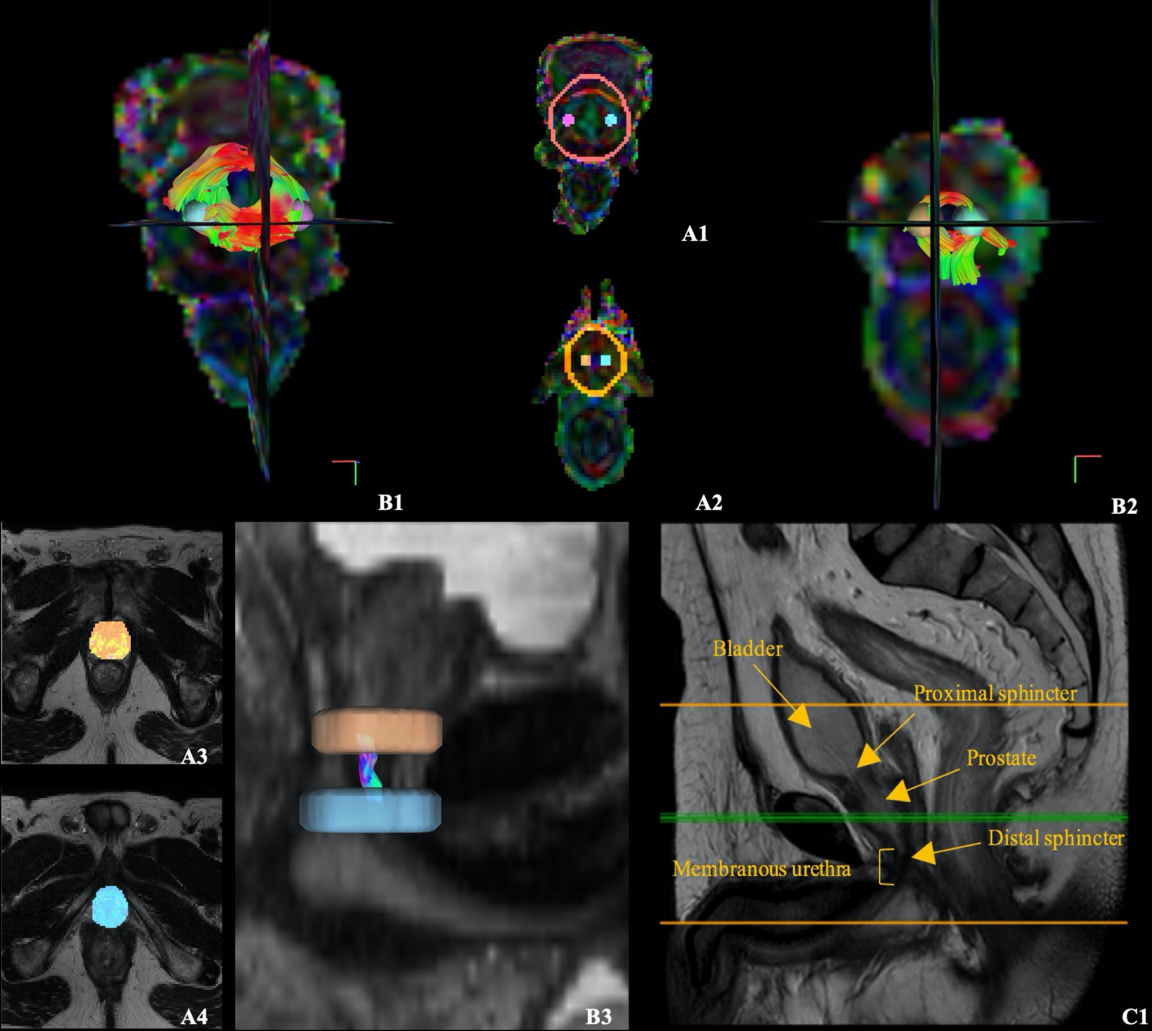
Axial views obtained from DSI Studio showing the region of interest (ROI) placement on the color-coded FA maps for the proximal sphincter (A1) and distal sphincter (A2), and on the T2-weighted image for the apex (A3) and bulb (A4) to track the membranous urethra. Fiber tracking results derived from the DTI images of an arbitrarily chosen study patient are shown for the proximal sphincter (B1), distal sphincter (B2), and membranous urethra (B3), color-coded based on the direction of the fibers—red for left–right (x), green for anterior–posterior (y), and blue for superior-inferior (z) directions. MRI T2-weighted image of a patient’s mid-sagittal slice labelled with the relevant anatomy, where the orange lines define the proximo-distal extent imaged in the DTI acquisition (C1)
